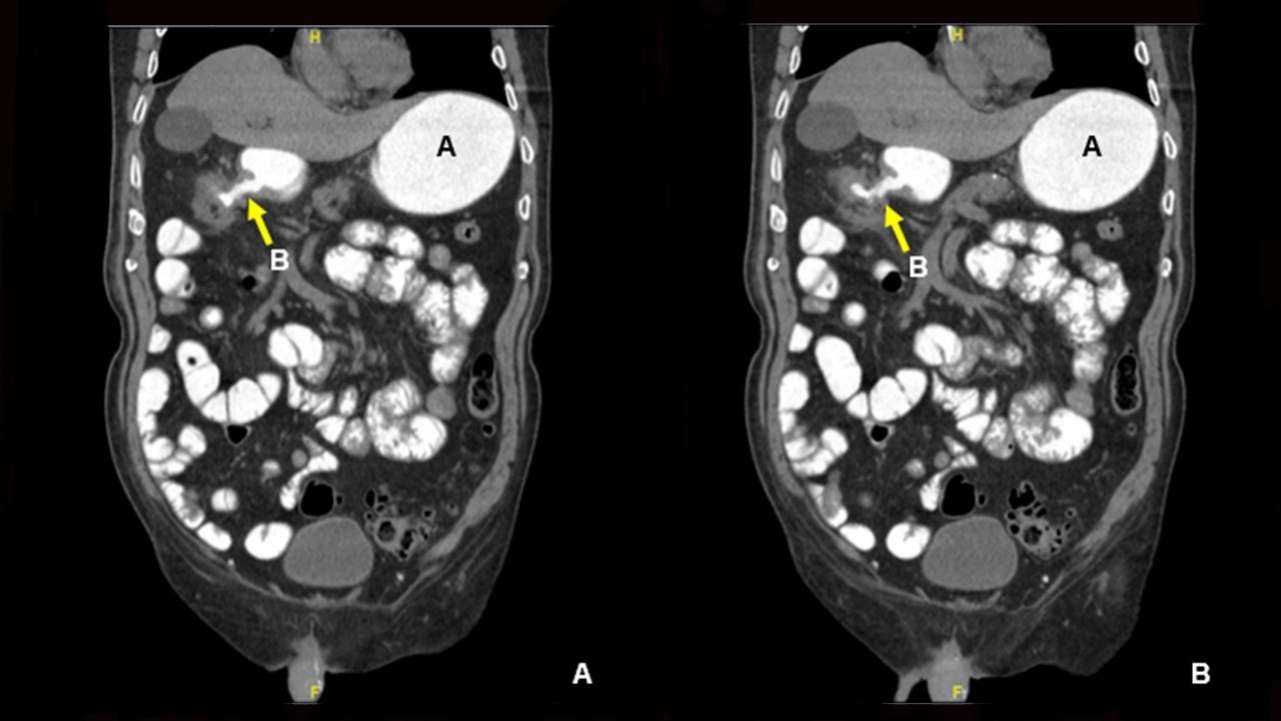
Coronal contrast CT scan images of abdomen demonstrates a dilated stomach (A) and gastrocolic fistula (B).
